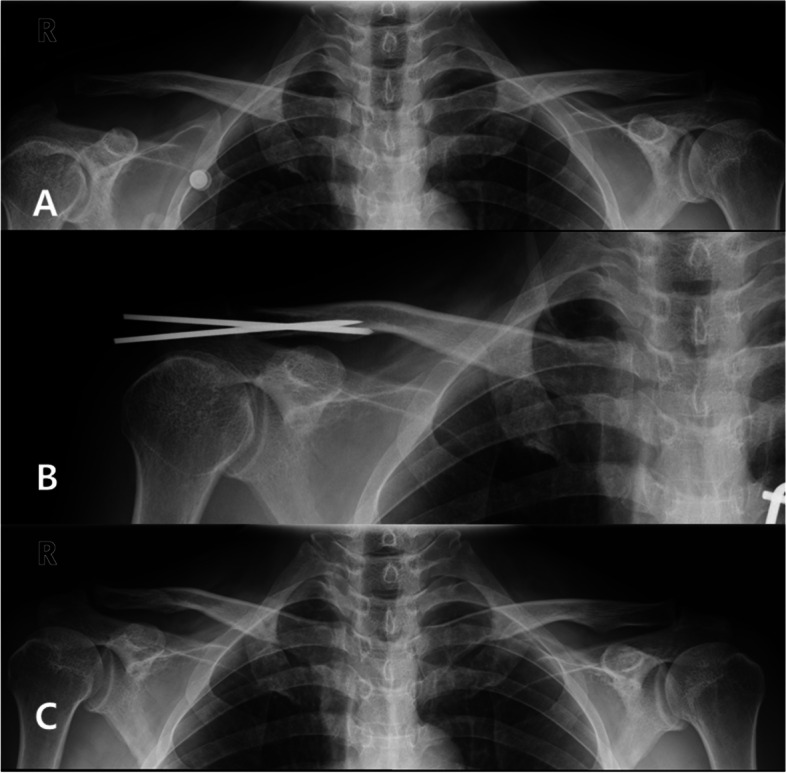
a Type V AC dislocation by Rockwood classification. b AC joint reduction and reconstruction of CC ligament with PLMT, and S-pins fixation. c The CC and AC distances were well maintained in the final follow-up X-ray
